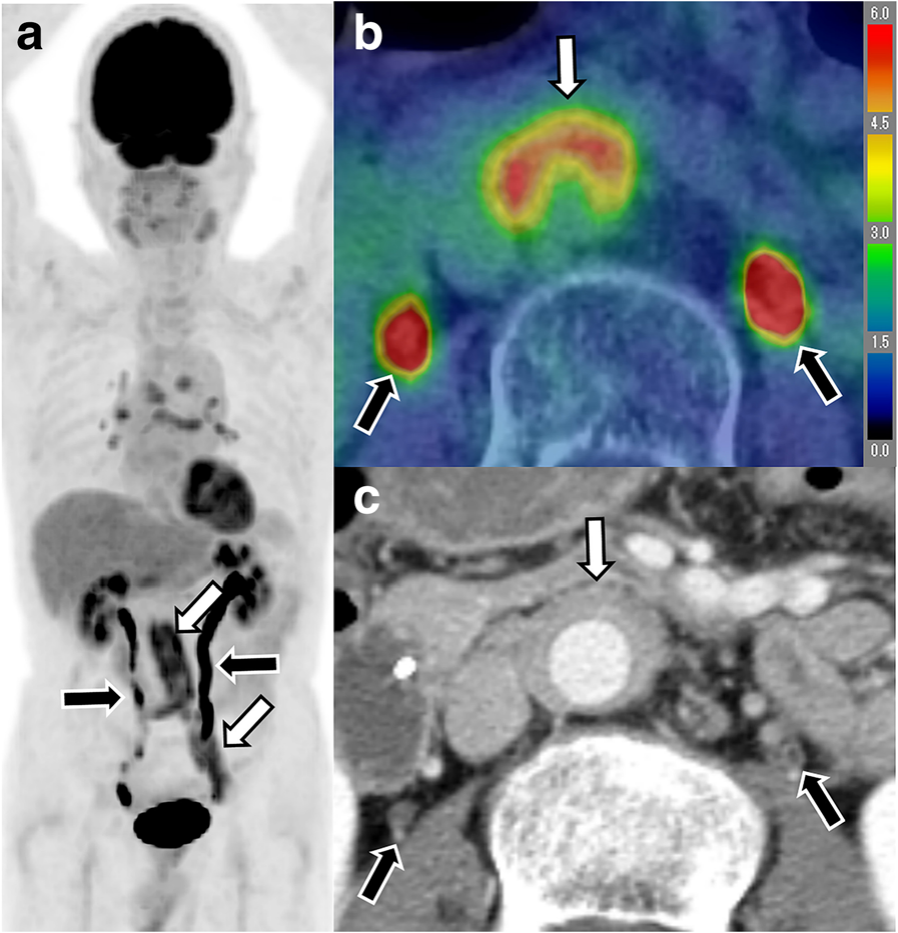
A 75-year-old man with involvement of the abdominal aorta (case #2). MIP image (a) showing multiple organ involvement, including hilar lymph node, abdominal aorta, and the left iliac artery (*white arrows*). FDG PET/CT fusion image (b) and CECT (c) exhibiting marked wall thickening of the abdominal aorta and abnormal FDG uptake (*white arrow*). Note the physiological uptakes in the bilateral urinary tract (*black arrows*)
